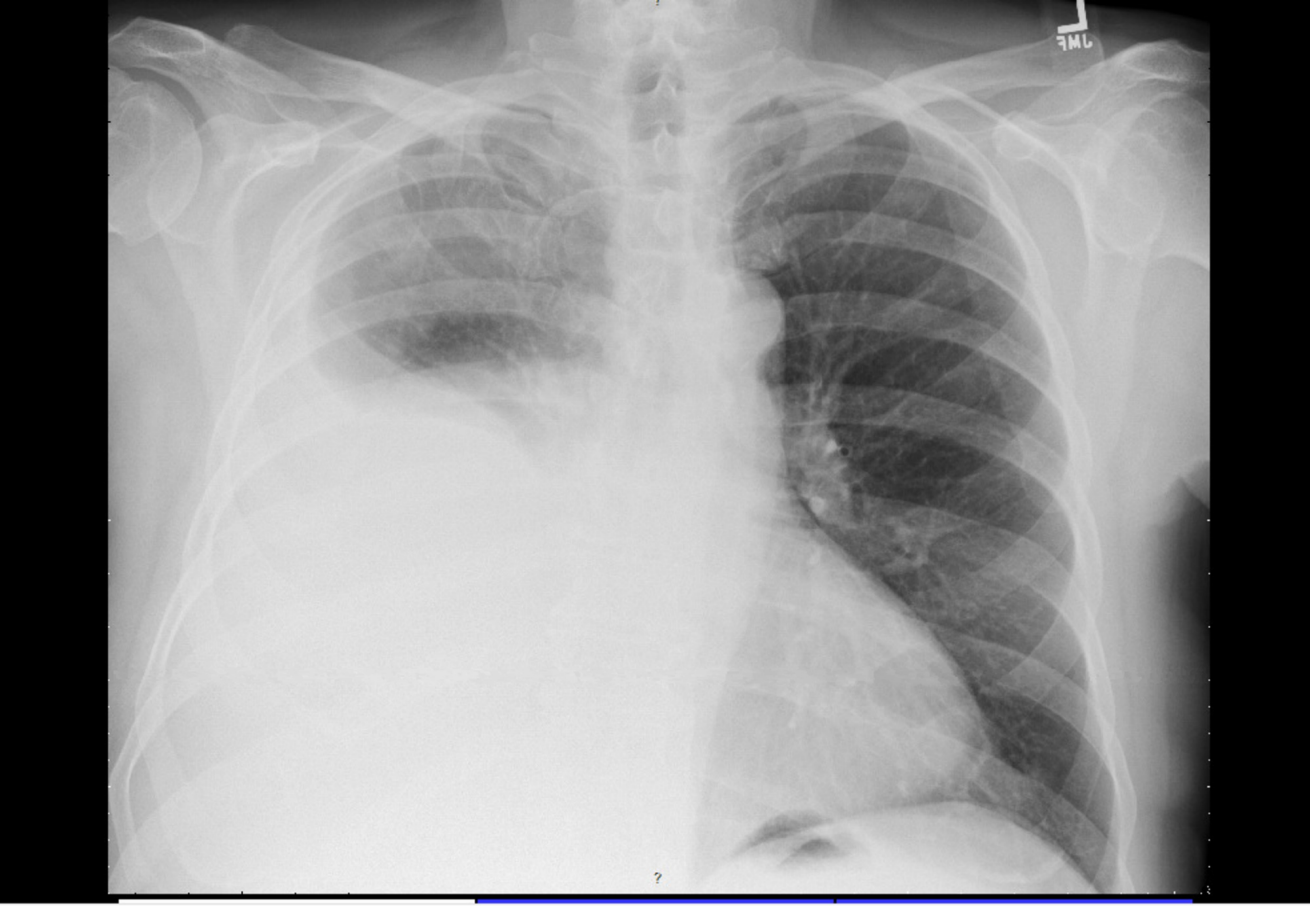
Chest X-ray demonstrating the right moderate-to-large pleural effusion.
